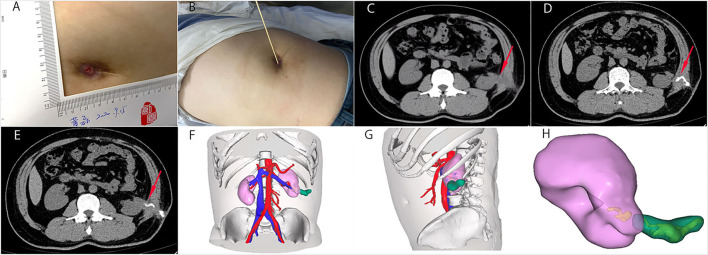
The general view and CT images of the nephrocutaneous fistula (NCF). (A,B) The fistula was 0.5 x 0.5 cm size and 6 cm deep on his left flank. (C) Abdominal CT showed the sinus tract (red arrow), extending from the surface of left kidney near the lower upper pole to the skin without renal/ureteral stone or hydronephrosis. (D,E) We injected contrast media into the sinus tract from the skin opening under pressure. The direction of the sinus tract is shown by the red arrow. (F–H) The three-dimensional reconstruction showed the relationship between the sinus tract and the left kidney. The sinus tract was marked green. We can see that the bottom of the sinus tract was closely connected with the lower pole of the left kidney.
